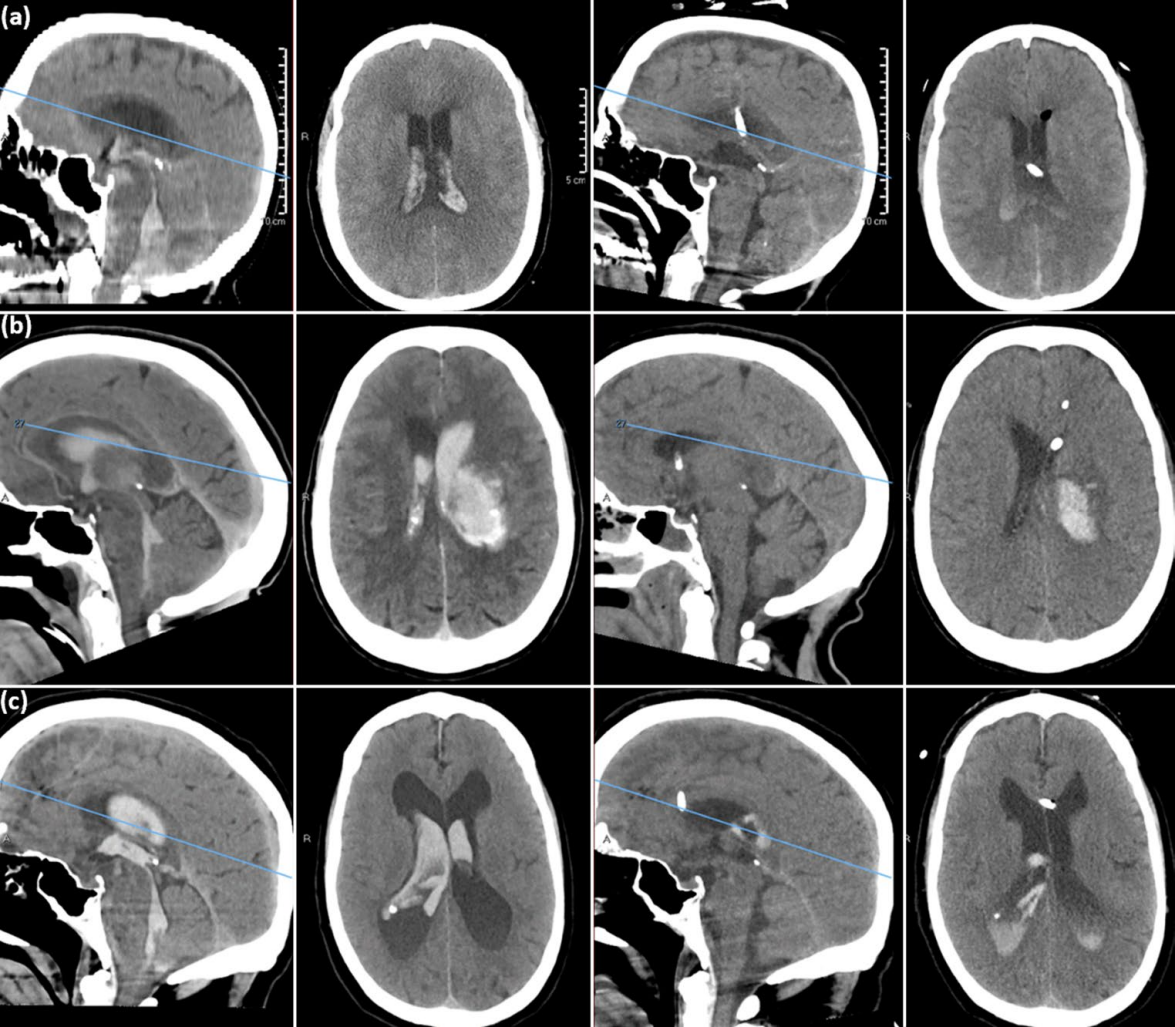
Sagittal and axial computed tomography (CT) images on admission (left) versus 72 h later (right). (a) case 1: PICA aneurysm rupture with casting of the fourth ventricle and cisterna magna; (b) case 2: Thalamic hemorrhage with ventricular casting; (c) case 3: IVH from choroidal aneurysm in moyamoya disease
